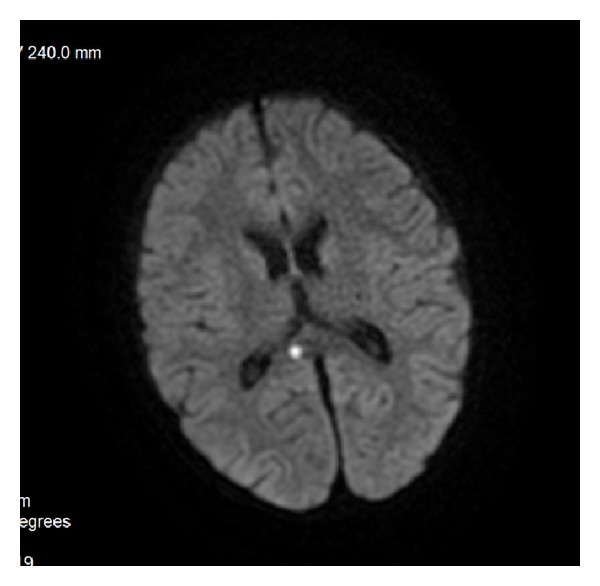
DWI sequence, axial plane. Focal infarct of the callosal splenium as a result of vasculitis in the course of streptococcal meningitis.
